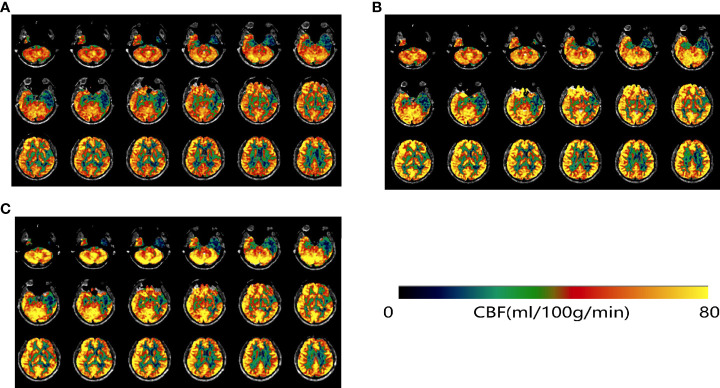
ASL-MRI on 9 February 2021 before GV-971 treatment (A), 29 July 2021 (B), and 18 January 2022 (C). After treatment with GV-971 (B, C), regions of low perfusion in green were visibly decreased.
